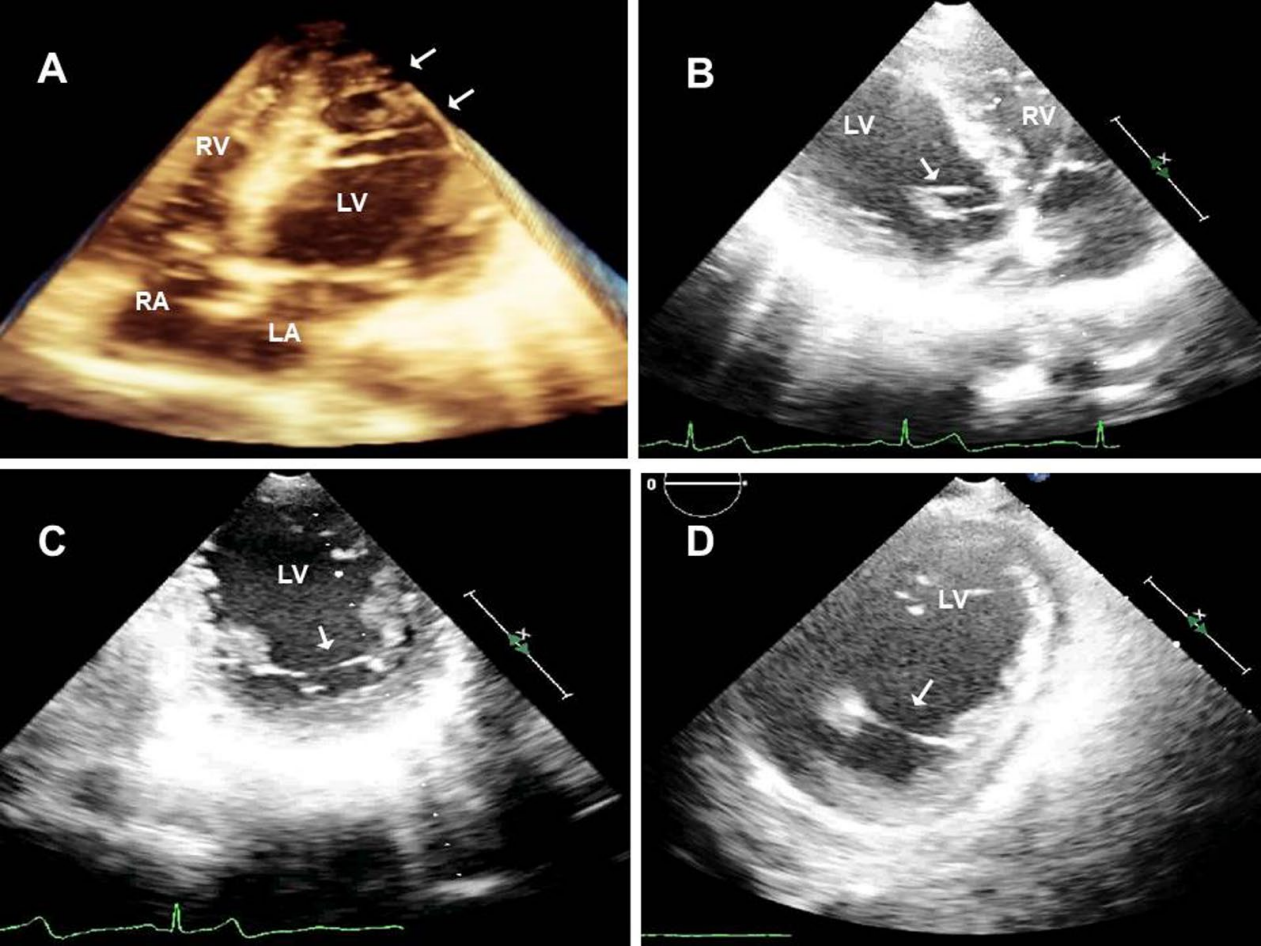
A Apical four-chamber three-dimensional transthoracic echocardiographic view with the appearance of multiple left ventricular false tendons (arrows). B Modified Four-chamber long-axis view depicting two left ventricular false tendons (arrow) from anterolateral mitral papillary muscle to basal segment of the anterior septal wall. Left ventricular false tendons are restricting the movement of the papillary muscle by pulling toward to interventricular septal wall. Fortunately, mitral valve dysfunction did not emerge secondary to these lesions. C, D Modified apical two-chamber view revealed complex branching left ventricular false tendons (arrow) between the base of anterolateral and posteromedial mitral papillary muscles and chordae tendinea
